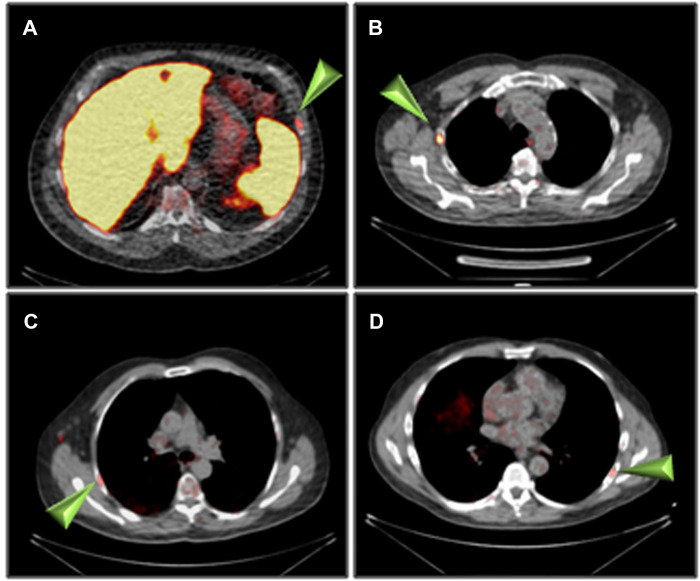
Rib uptake of [^18^F]PSMA-1007 (arrowheads) 2 h after injection. (A) patient #4, (B) patient #6, (C) patient #8, (D) patient #11.
